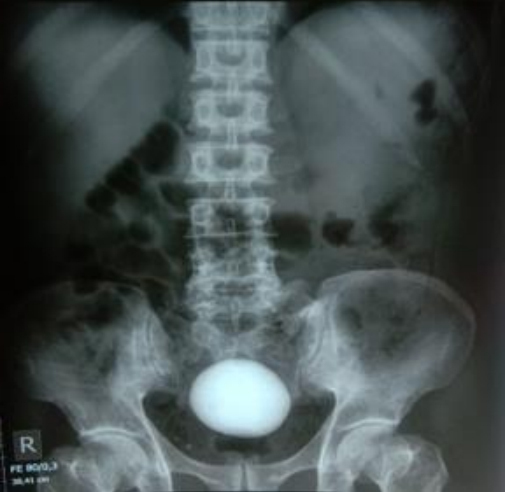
The patient’s bladder stone seen on an X-ray.
